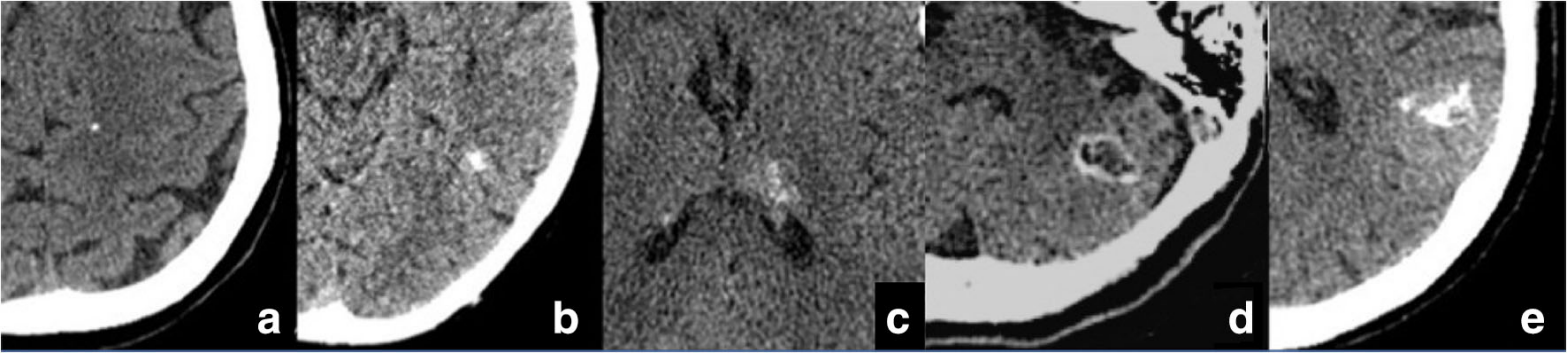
Types of calcifications (pre-contrast CT scan): punctate (a), nodular (b), cluster (c), ring (d), and coarse (e)
